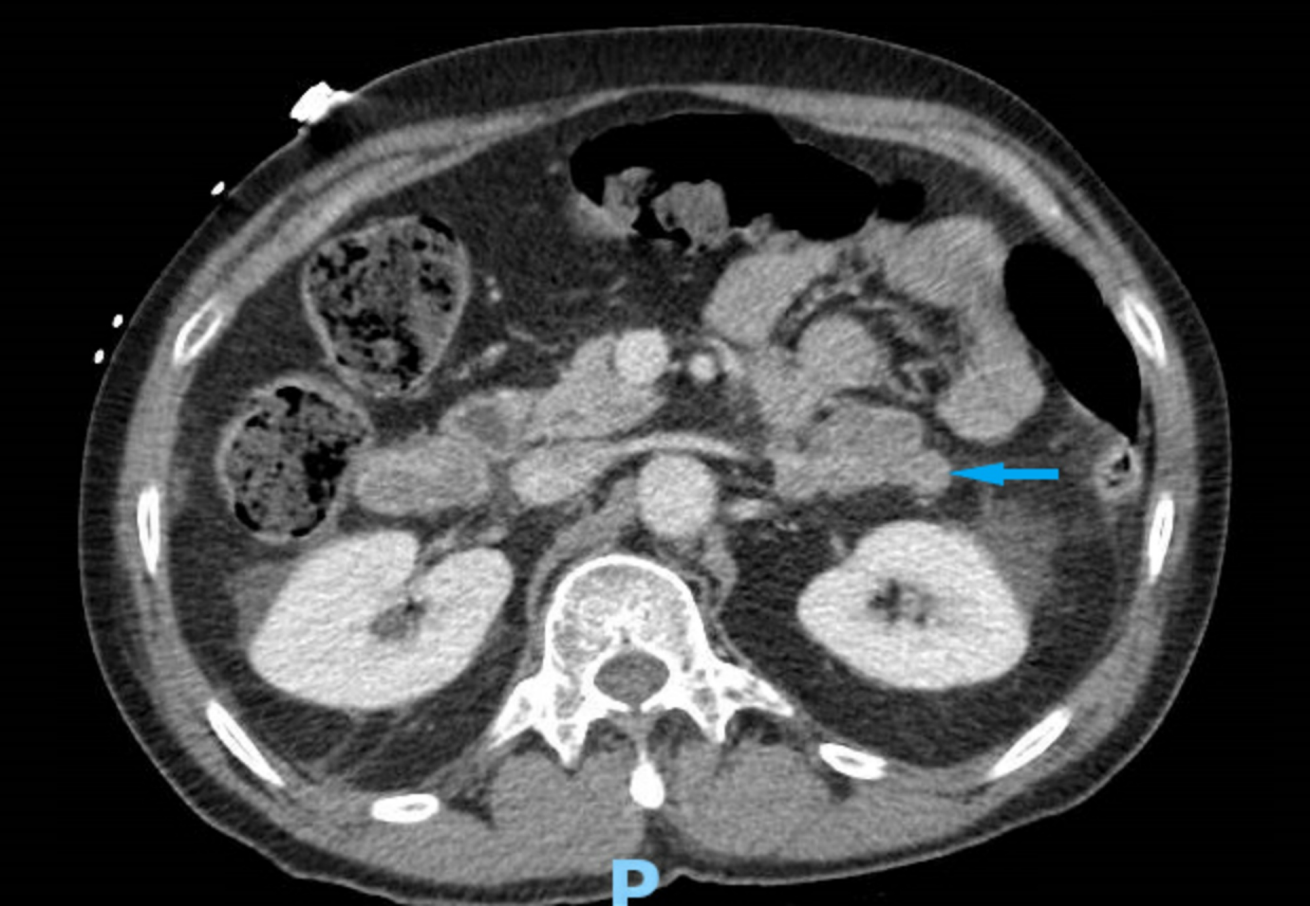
Computed tomography of the abdomen and pelvis showing bilateral adrenal nodules
